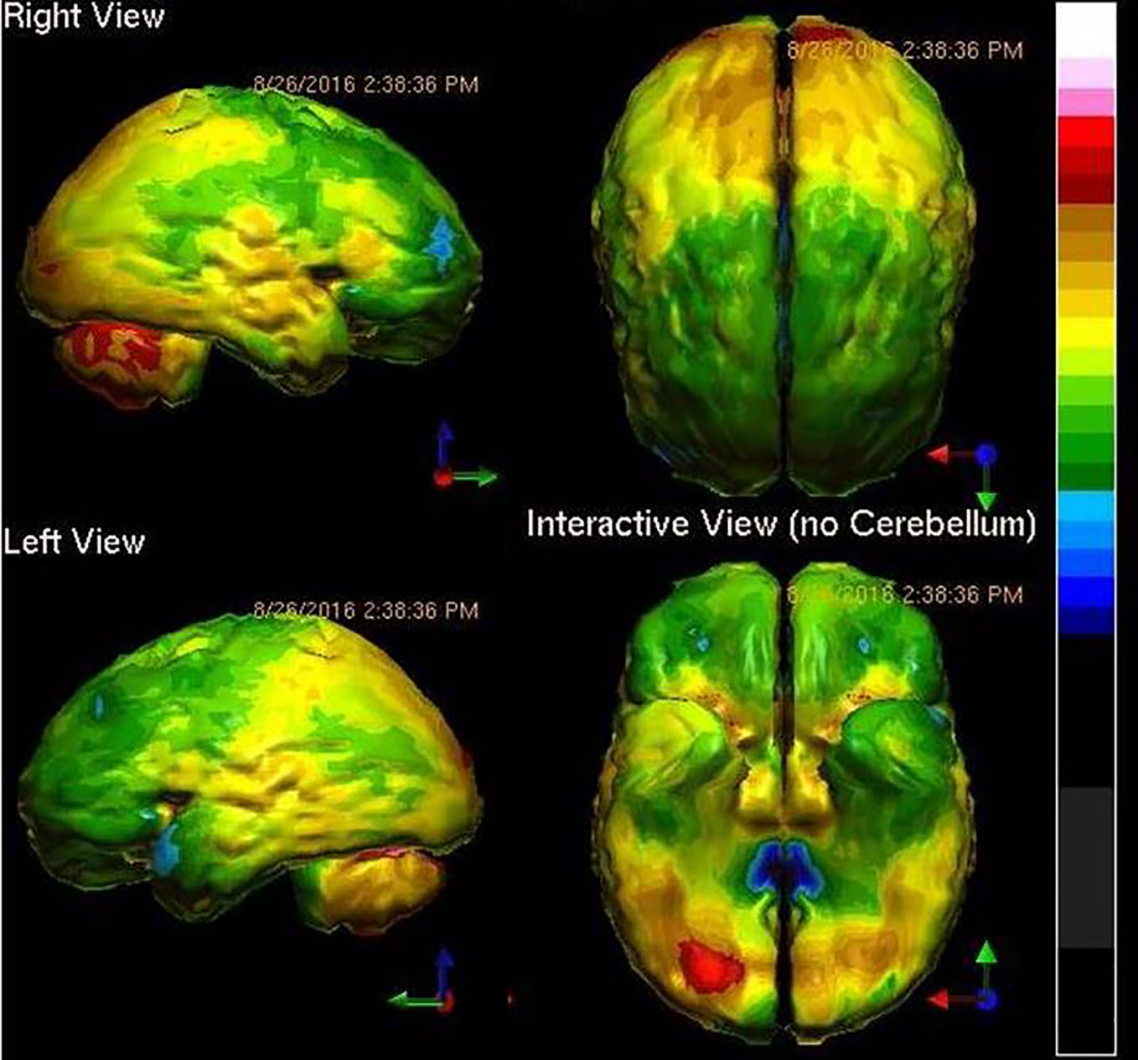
Tc-99m-HMPAO perfusion SPECT scan data presented in surface rendering. The color scale is as in Figure 1. Bilateral frontal and temporal hypoperfusion (green and blue) is clearly evident and is consistent with traumatic brain injury.
